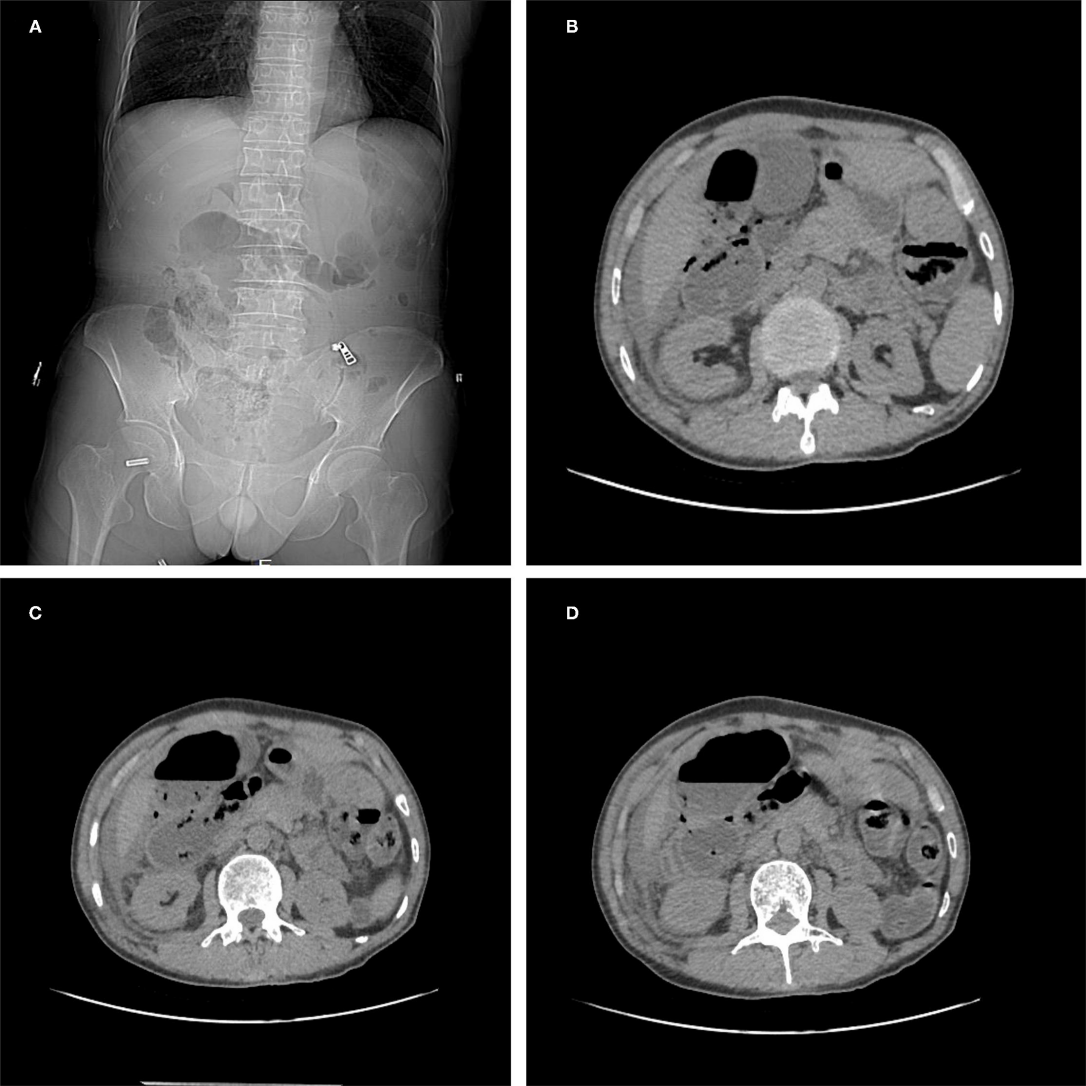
Abdominal CT examination (A–D): a small amount of free gas in the abdominal cavity, ascites, gastrointestinal perforation.
